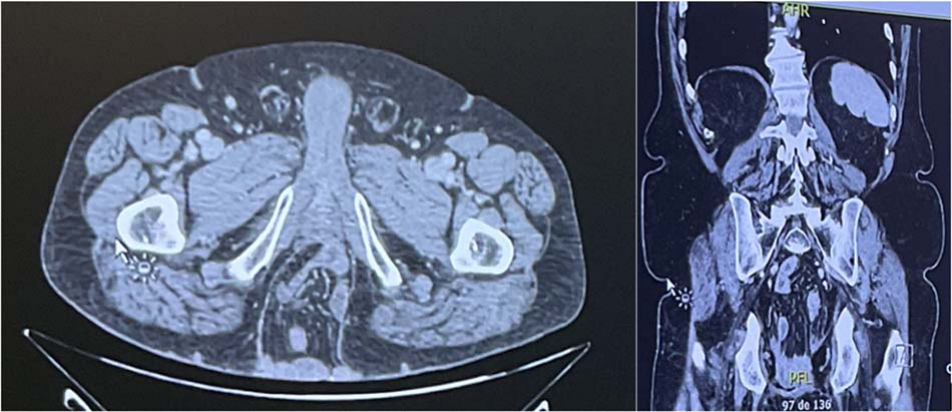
CT scan revealing a PH of 9.5 × 8.7 × 15 cm, containing small bowel.
